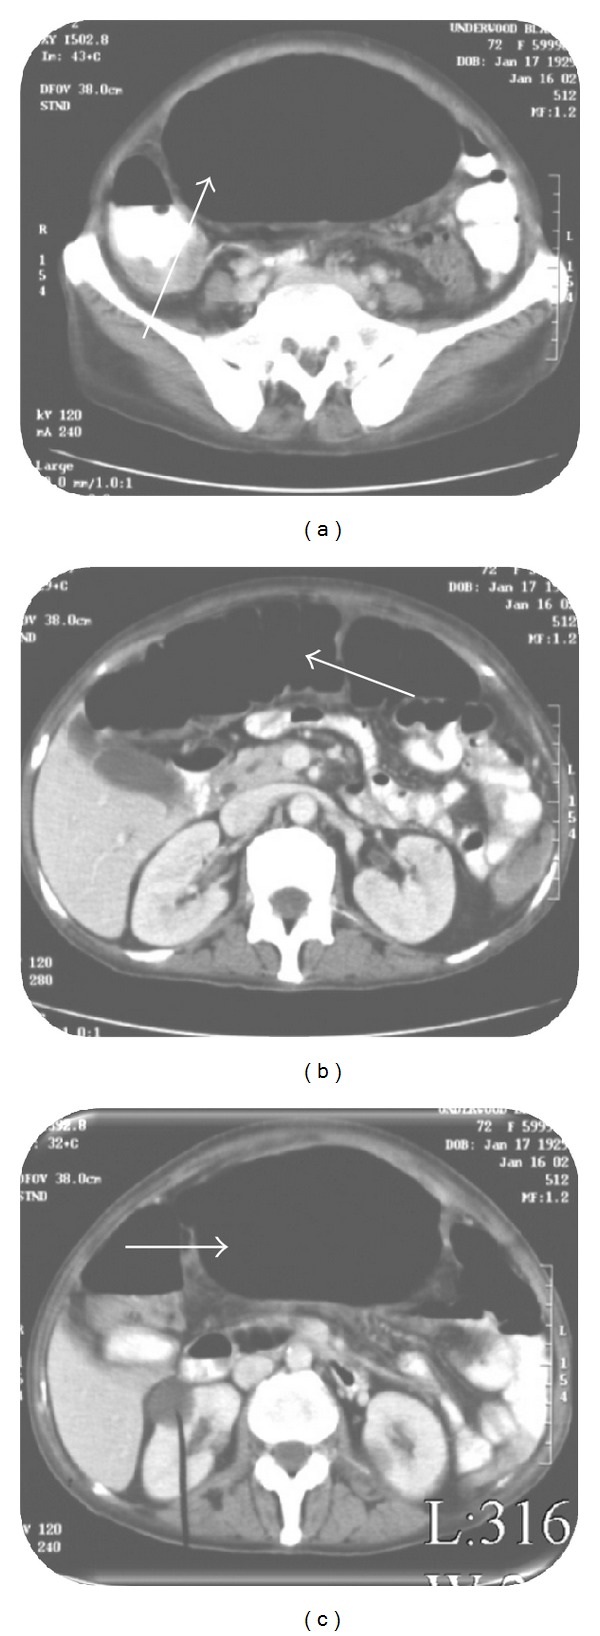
((a), (b), and (c)) Abdominal CT showing air fluid level with a dilated loop of sigmoid colon.
